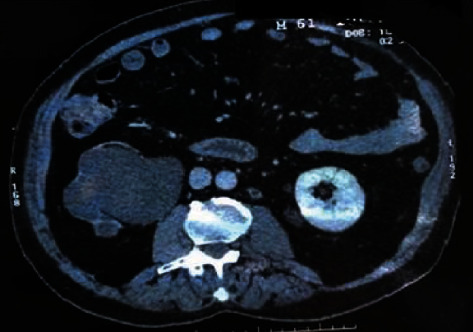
CT of June 2, 2016 showing posteromedial oval image to the left kidney of 2,4 cm.
